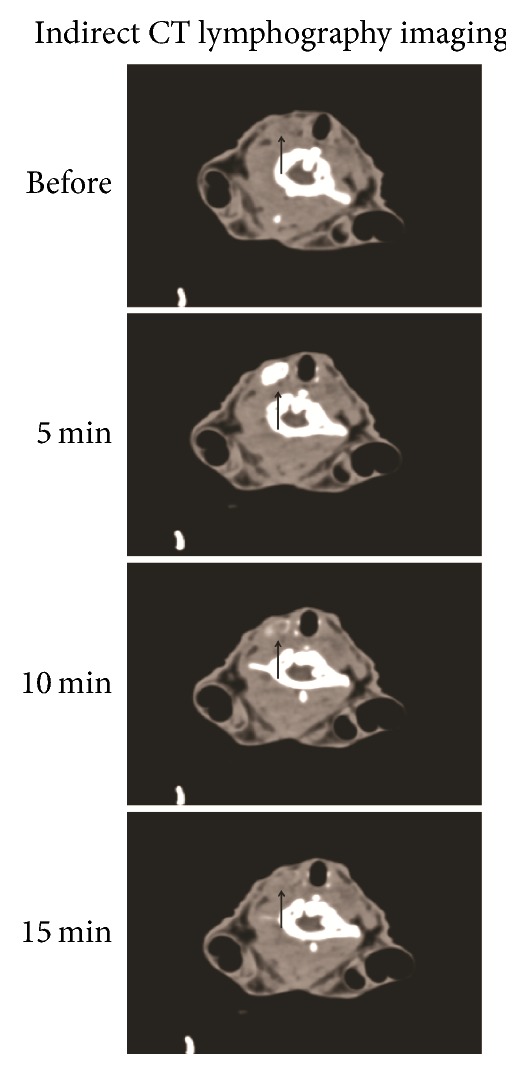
The CT imaging of SLN (black arrow, deep cervical lymph node) before the injection and 5, 10, and 15 minutes after injection of the mixture of Omnipaque and the nanocarbon particle (0.5 mL, volume ratio = 1 : 1) into the right ventrolateral submucosa of the tongue.
